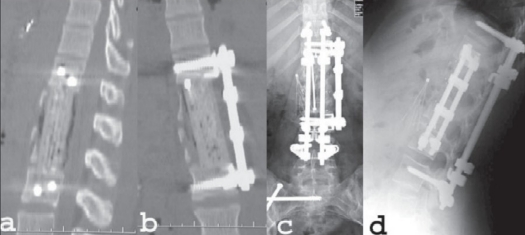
Sagittal reconstruction of a CT scan (a) of the same patient as fig 1.1 & 1.2 demonstrating good positioning of the stackable carbon fiber cage with complete decompression of the spinal canal. Kaneda screws are seen at T12 and L3. Coronal reconstruction of a CT (b) scan demonstrating good positioning of the stackable carbon fiber cage and the Kaneda screw-rod fixation device. AP plain film radiograph (c) obtained two years after surgery demonstrates solid fusion and excellent alignment. Lateral plain film radiograph (d) at two years postoperatively demonstrates preservation of sagittal alignment. At five years post-injury, the patient was neurologically intact with normal bowel and bladder function. She was gainfully employed and despite a solid T11-L4 fusion, she was able to forward bend and touch her toes with her knees fully extended. She required no pain medications
